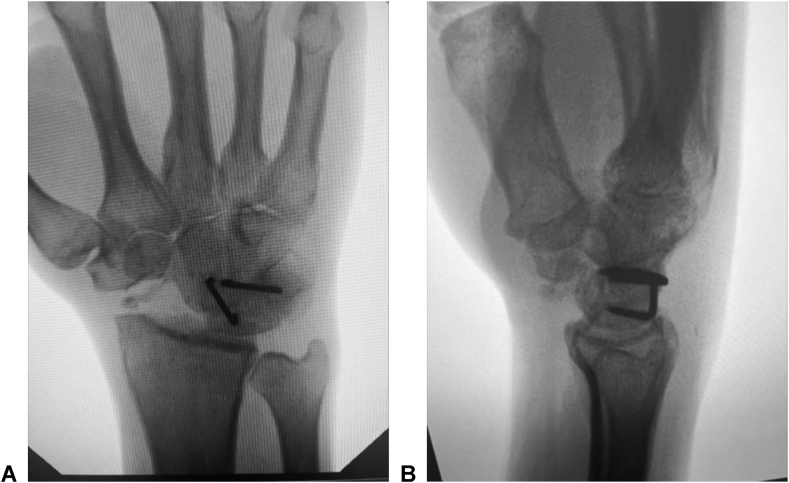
A Posterior-anterior X-ray of wrist after completion of four-corner fusion using this construct. B Lateral X-ray of wrist after completion of four-corner fusion using this construct.
